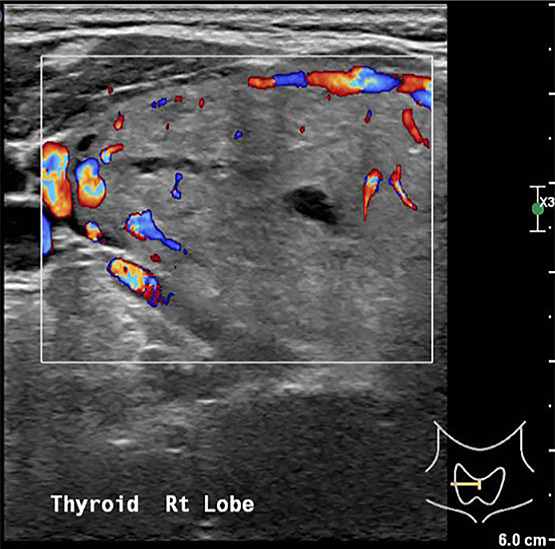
Ultrasound scan of right thyroid lobe
